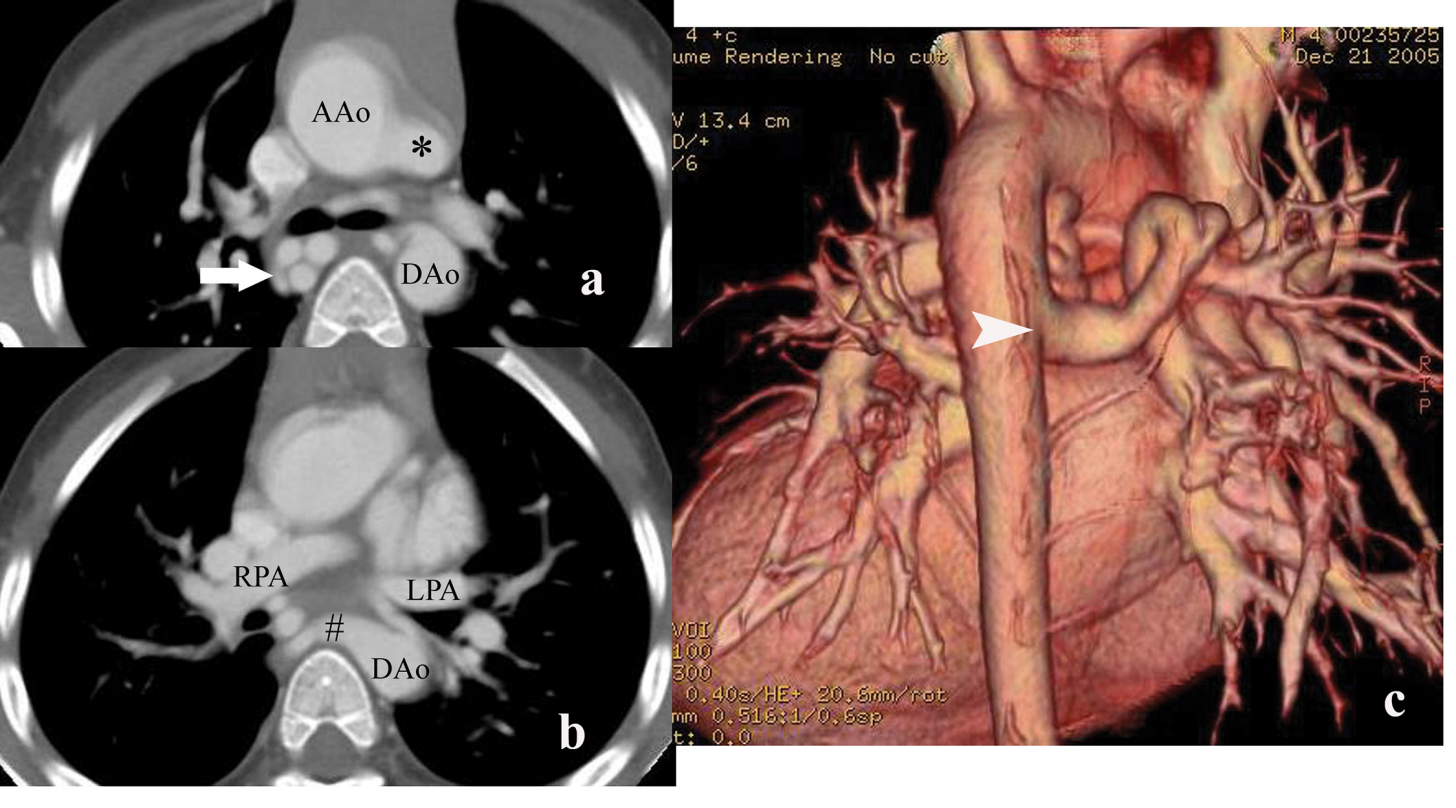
MDCT in a 4-year-old boy with PA-VSD. (a) Axial CT shows the patients has patent ductus arteriosus(*). Some MAPCAs (white arrow) from the descending aorta (DAo) to the right lung. (b) Axial CT demonstrates good-sized right pulmonary artery (RPA) and left pulmonary artery(LPA). The orifice of a MAPCA (#) is depicted clearly. (c) 3D Volume-rendered image (posterior view) shows a MAPCA (white arrowhead) from the descending aorta to the right lung.
